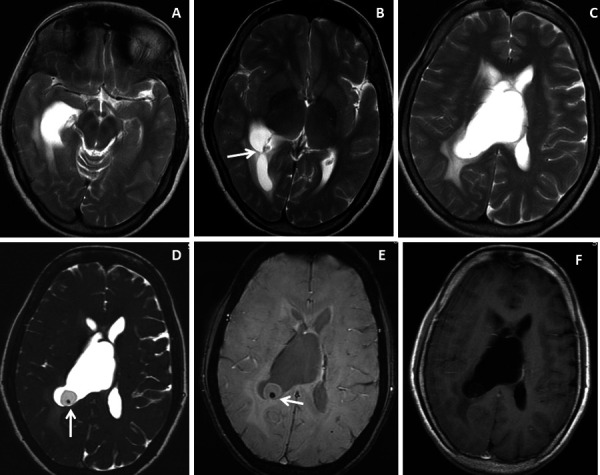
Brain magnetic resonance imaging of 52 years female with headache and vomiting. (A-C) Axial T2WI images show asymmetrical hydrocephalus with entrapment of temporal horn of the right lateral ventricle with narrowing / adhesion in the trigone of the lateral ventricle (arrow) with surrounding periventricular CSF seepage. (D) Heavily T2-weighted image shows an intraventricular neurocysticercus lesion with a T2 hypointense scolex within (arrow). (E) Axial susceptibility-weighted image shows nodular blooming of the calcified scolex within the thin wall IVNCC (arrow). (F) Axial post-gadolinium T1W image shows thin smooth peripheral cyst wall enhancement with the smooth ependymal enhancement of the asymmetrically dilated lateral ventricle.
